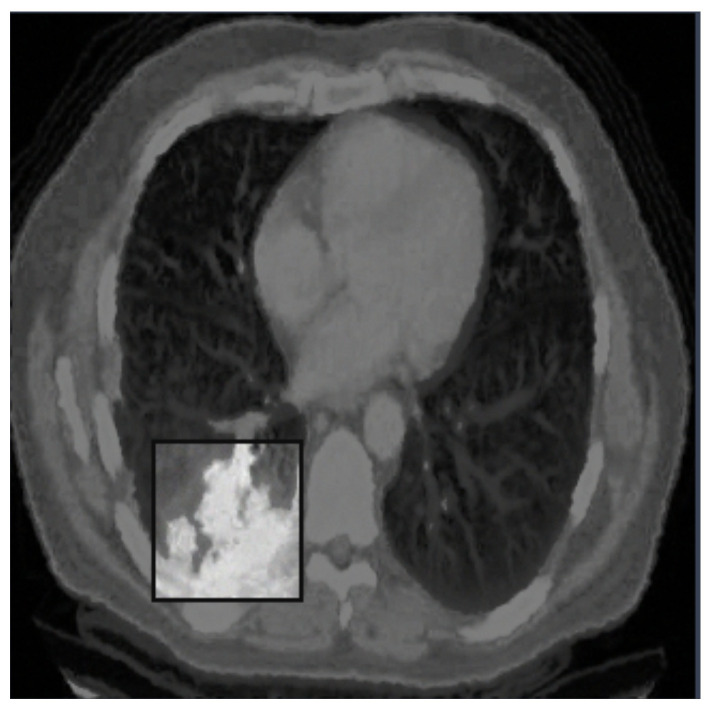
An example of a CT image belonging to the analyzed dataset, with the annotation related to adenocarcinoma.
